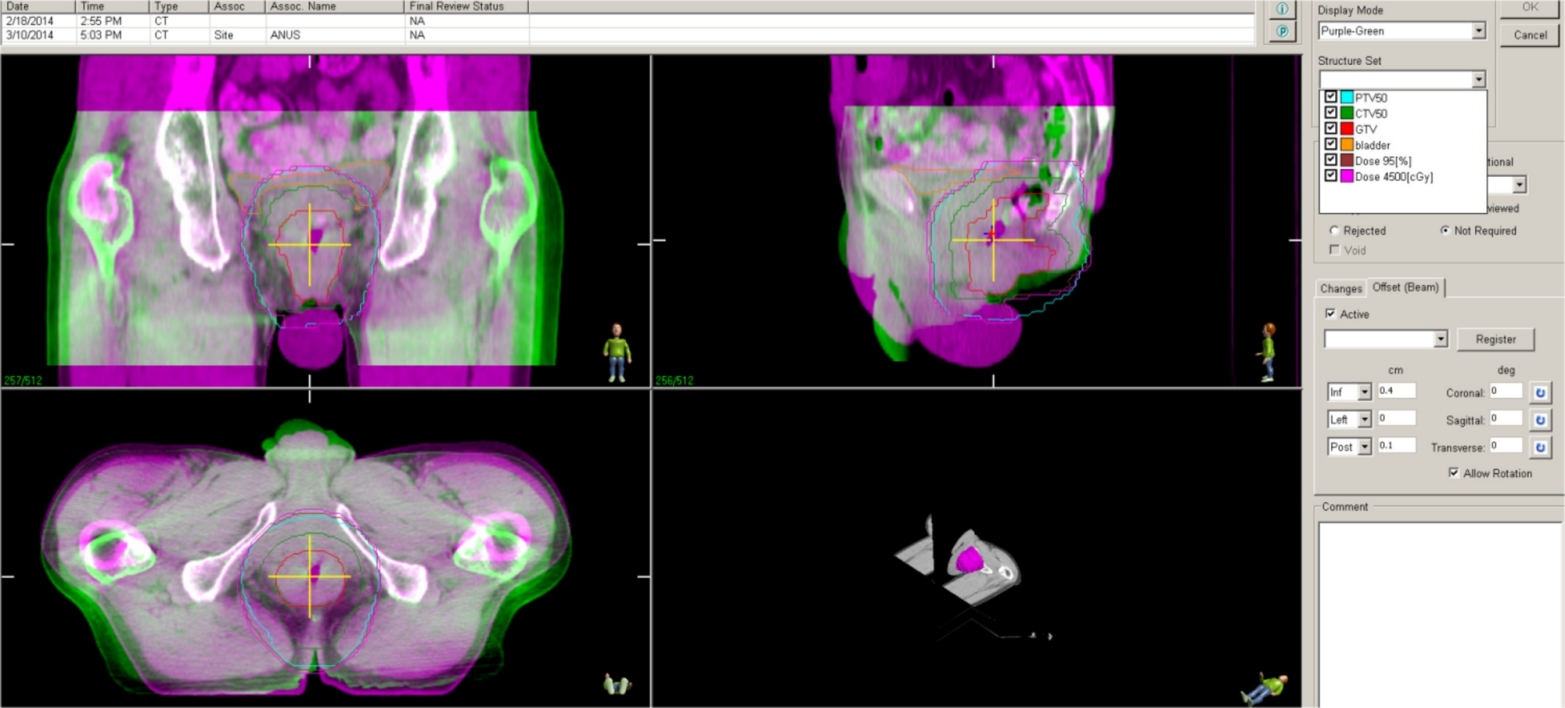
Daily CBCT in green overlapping with planning CT in purple Note Beam Offset of only 0.4 cm, 0 cm and 0.1 cm to the inferior, left and posterior, respectively, which will be auto-matched to pelvic bone. CBCT, cone beam computed tomography; CT, computed tomography; PTV50, planning target volume to receive 50Gy; CTV50, clinical target volume to receive 50Gy; GTV, gross tumor volume
